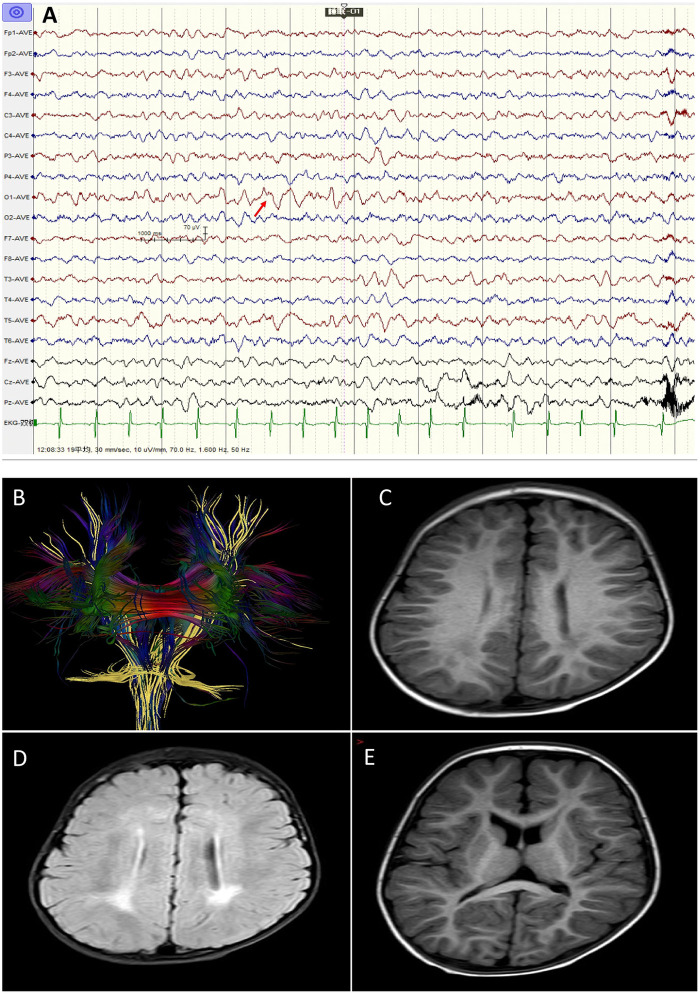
Neuroimaging and electroencephalographic findings (A) electroencephalography (EEG) showing focal epileptiform discharges (indicated by the red arrow) originating from the right occipital lobe. (B) DTI demonstrating sparse bilateral white matter tracts. (C–E) Axial MRI showing delayed myelination of the bilateral cerebral white matter, predominantly in the periventricular regions.
